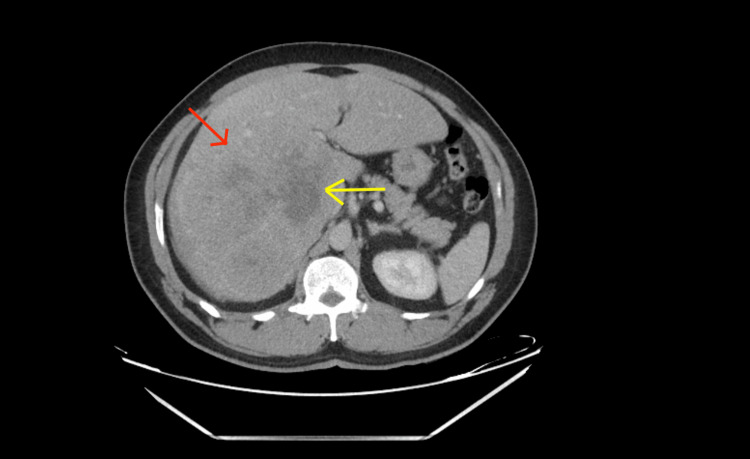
Triple-phase CT with liver mass protocol (venous phase) A decreased enhancement of the capsule around the mass (red arrow) is seen with a further low enhancement of the mass (yellow arrow) as the rest of the liver is receiving blood supply containing the contrast from the portal vein.
